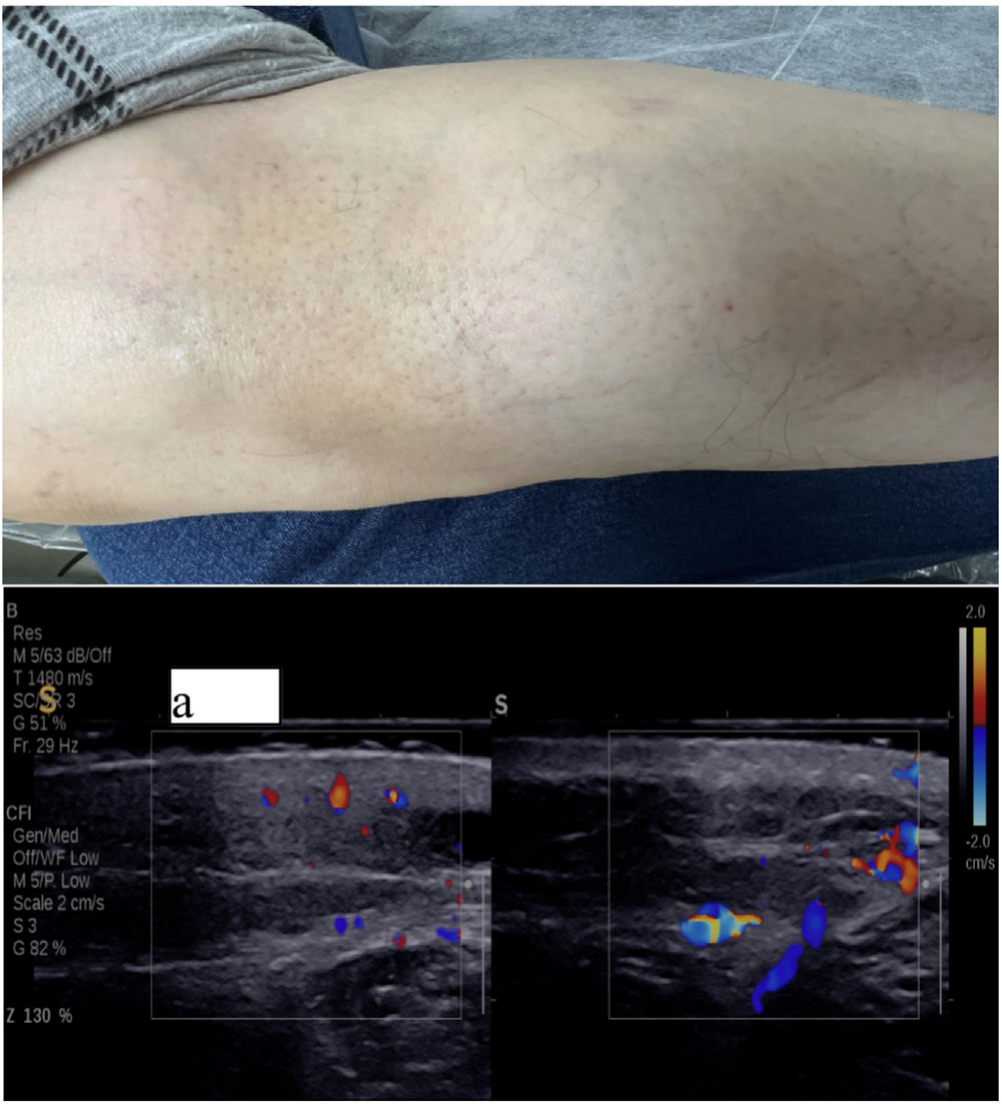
Color Doppler ultrasound images of morphea plaque in infrapatellar subcutaneous region of 50‐year‐old male showing dermal thickening and hypoechogenicity, as well as increased echogenicity of hypoderm causing dermal‐hypodermal blurredness and increased subcutaneous vascularity comparing adjacent normal soft tissue in favor of active plaque.
